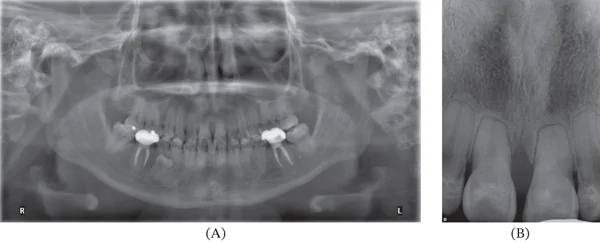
(A) Case #2 panoramic radiograph long‐term follow‐up. (B) Case #2 periapical radiograph long‐term follow‐up.
